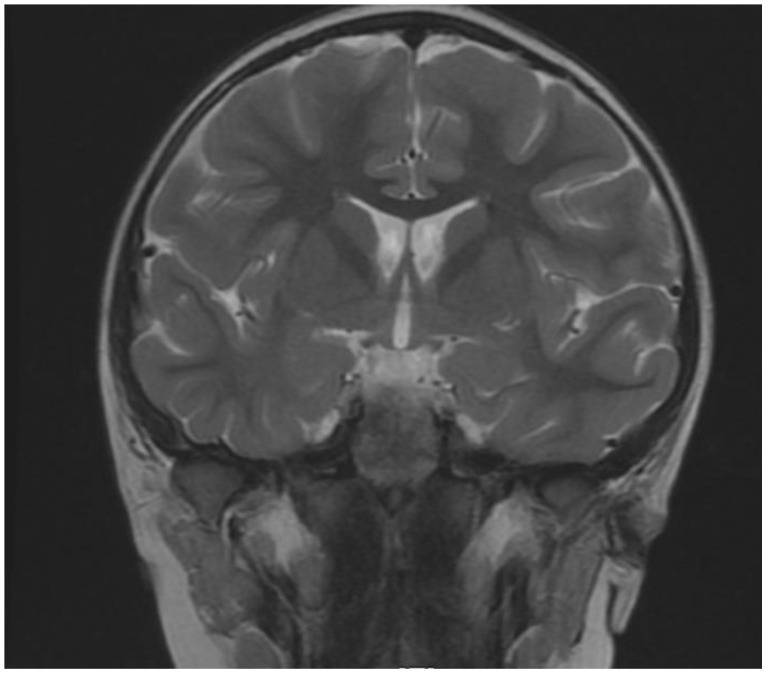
Coronal (T2-weighted sequence). Slight delayed myelination.
